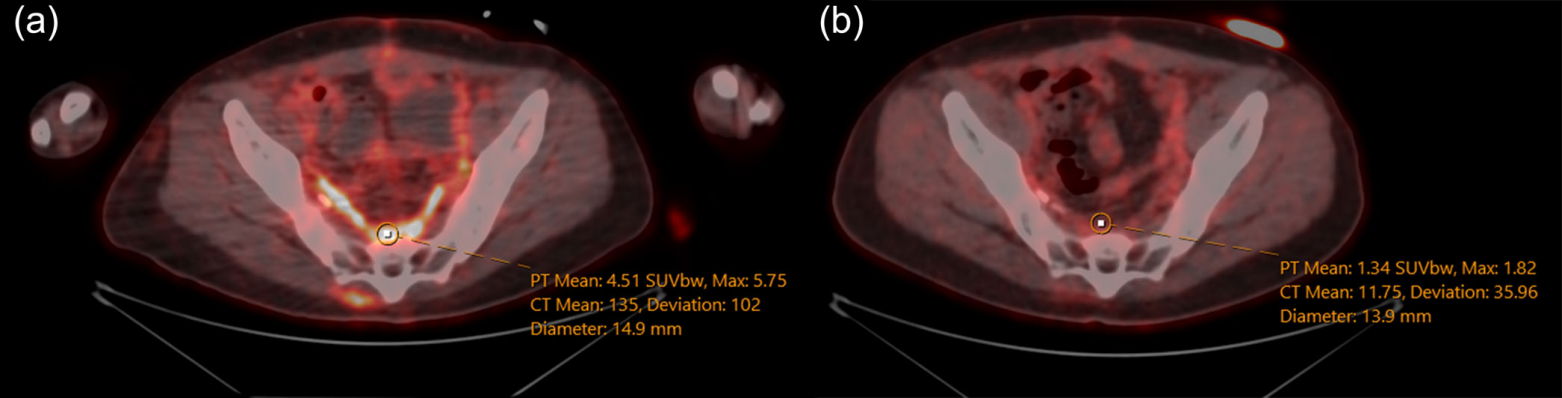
(a) PET/CT fusion axial view performed 1 month postoperatively demonstrated high FDG avidity in the shape of the placed bio‐absorbable mesh. (b) PET/CT fusion axial view performed 9 months postoperatively showing significantly decreased FDG avidity.
